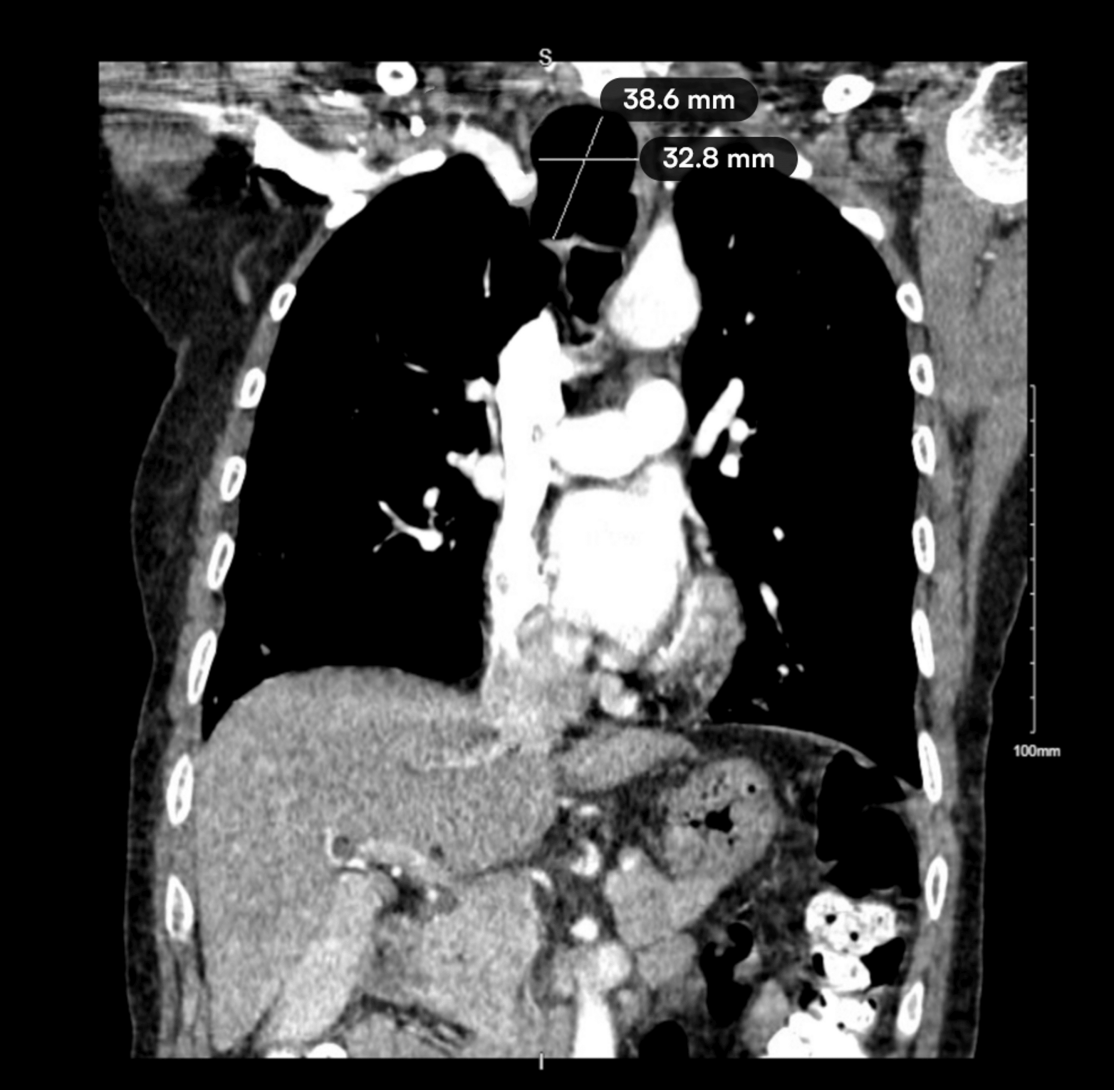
CT scan displaying a 38.6x32.8 mm tracheocele along the tracheal wall
